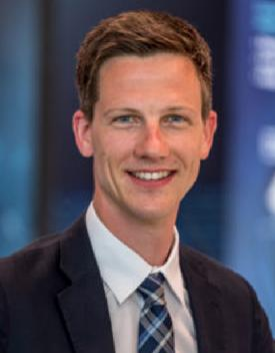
Senior Physician and interventional cardiologist at the University Heart Center Freiburg - Bad Krozingen, Germany. Certified with the highest degree of Cardiac-MRI and Cardiac-CT of the German Cardiac Society. Chair of Young DGK.
